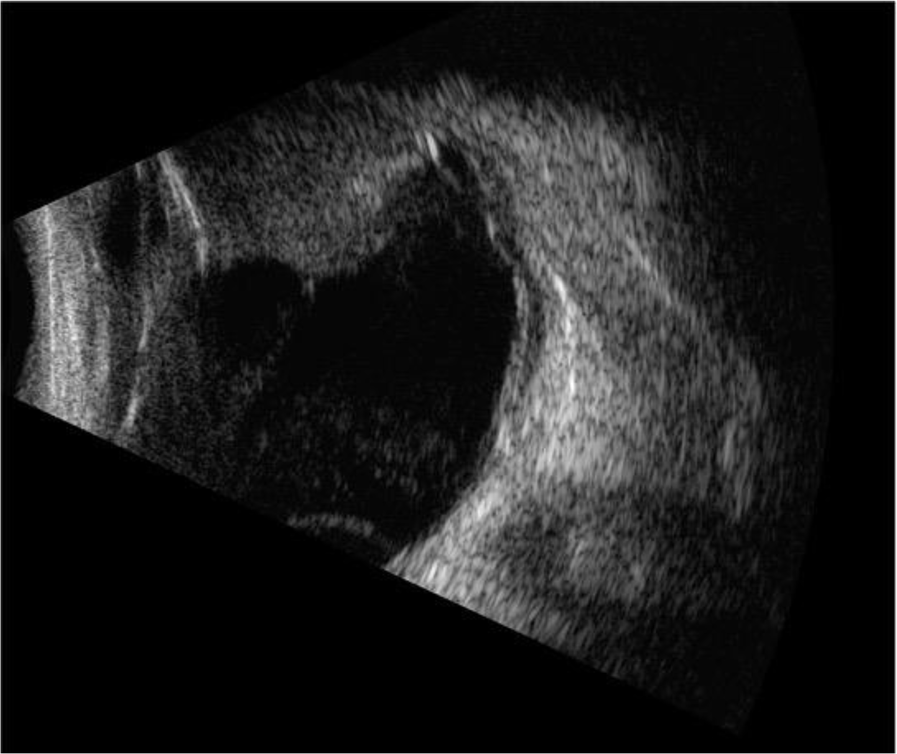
B-scan ultrasonograpy of right eye before diagnosis vitrectomy showed vitreous opacity and retinal detachment with ring occupying lesion of ciliary body
